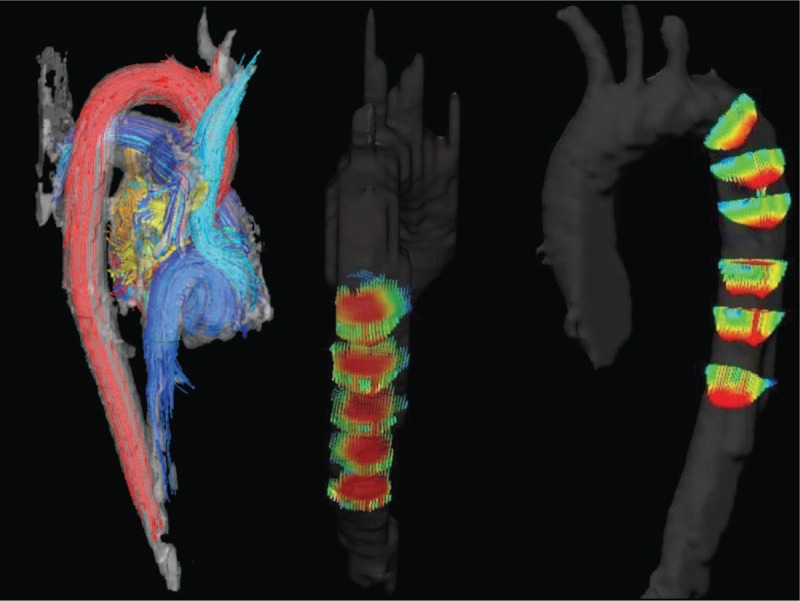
4D-MRI velocity mapping indicates that faster flow (red) is more inclined externally and proximal to the middle of the thoracic descending aorta (images courtesy of K. Itatani). 4D-MRI = 4-dimensional magnetic resonance imaging.
